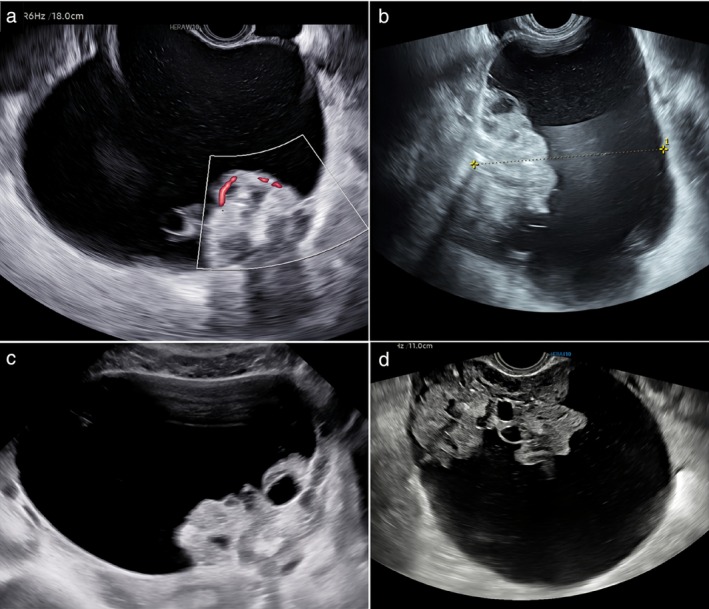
Color Doppler (a) and grayscale (b,c,d) ultrasound images of four ovarian immature teratomas. Using pattern recognition, these tumors were described as cystic with a solid component protruding into the cyst cavity like a large papillary projection. Solid components show pathognomonic echogenicity, with hyperechogenic areas, cysts and shadowing.
